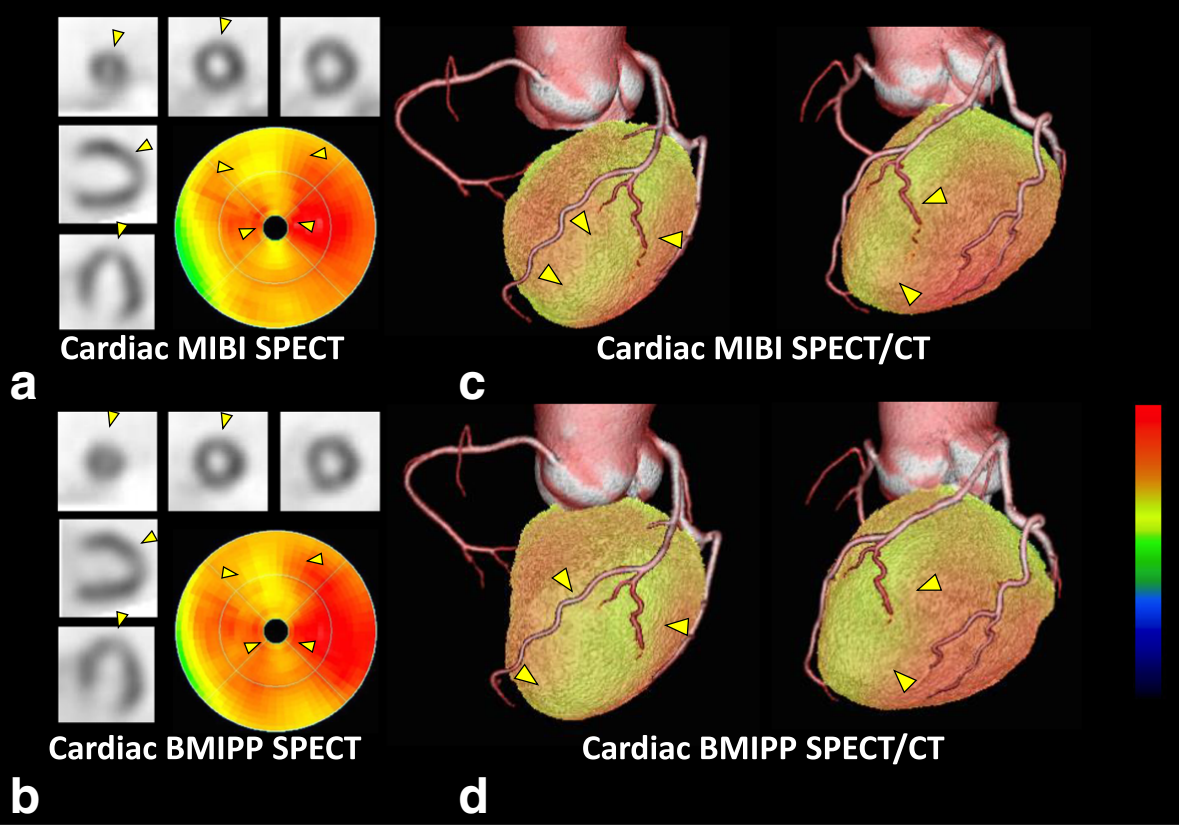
60-year-old woman with ACS. Cardiac MIBI and BMIPP SPECT images show accumulation defects in the mid-distal anteroseptal to apical wall and this patient may have ACS in the LAD territory (a, b). SPECT/CT fused images revealed that the accumulation defect is congruent with first diagonal branch territory, confirming ACS due to coronary vasospasm (c, d)
